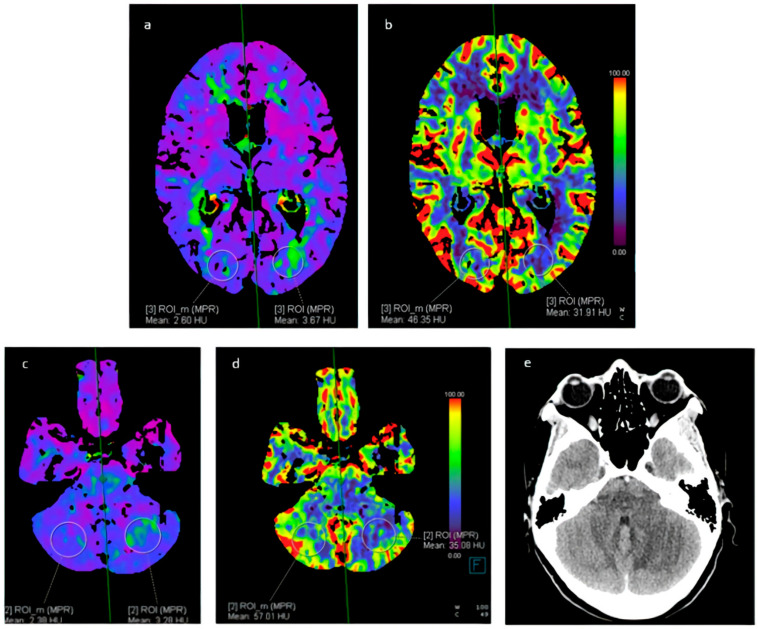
A 37-year-old female with cocaine-induced RCVS, admitted to the Emergency Department due to headache and bilateral hypovision. Perfusion CT showed (a,b) an increased TMAX and reduced CBF in the left occipital lobe; (c,d) an increased TMAX and a reduced CBF in the left cerebellar hemisphere. (e) CT showed a cerebral infarct on the left cerebellar hemisphere corresponding to the area indicated on perfusion CT. RCVS: reversible cerebral vasoconstriction syndrome. TMAX: time-to-maximum. CBF: cerebral blood flow.
